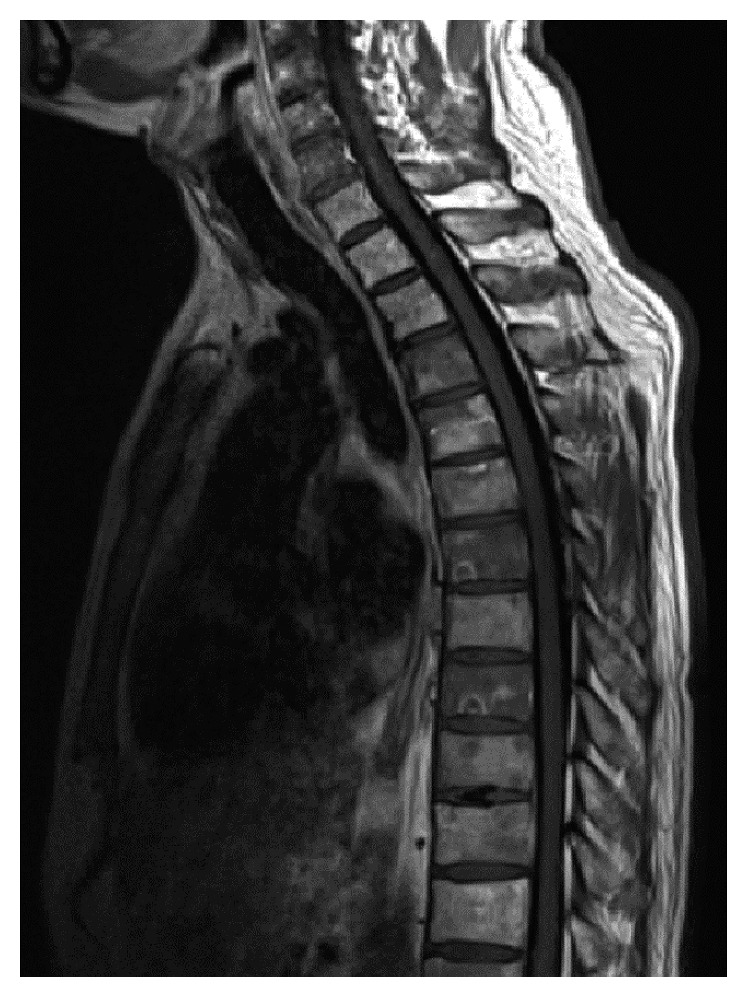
MRI of the thoracic and lumbar spine: compression vertebral fracture at L4 with retropulsion of the posterior vertebral body causing severe spinal stenosis. Extraosseous extension of tumor into ventral epidural space at T12.
